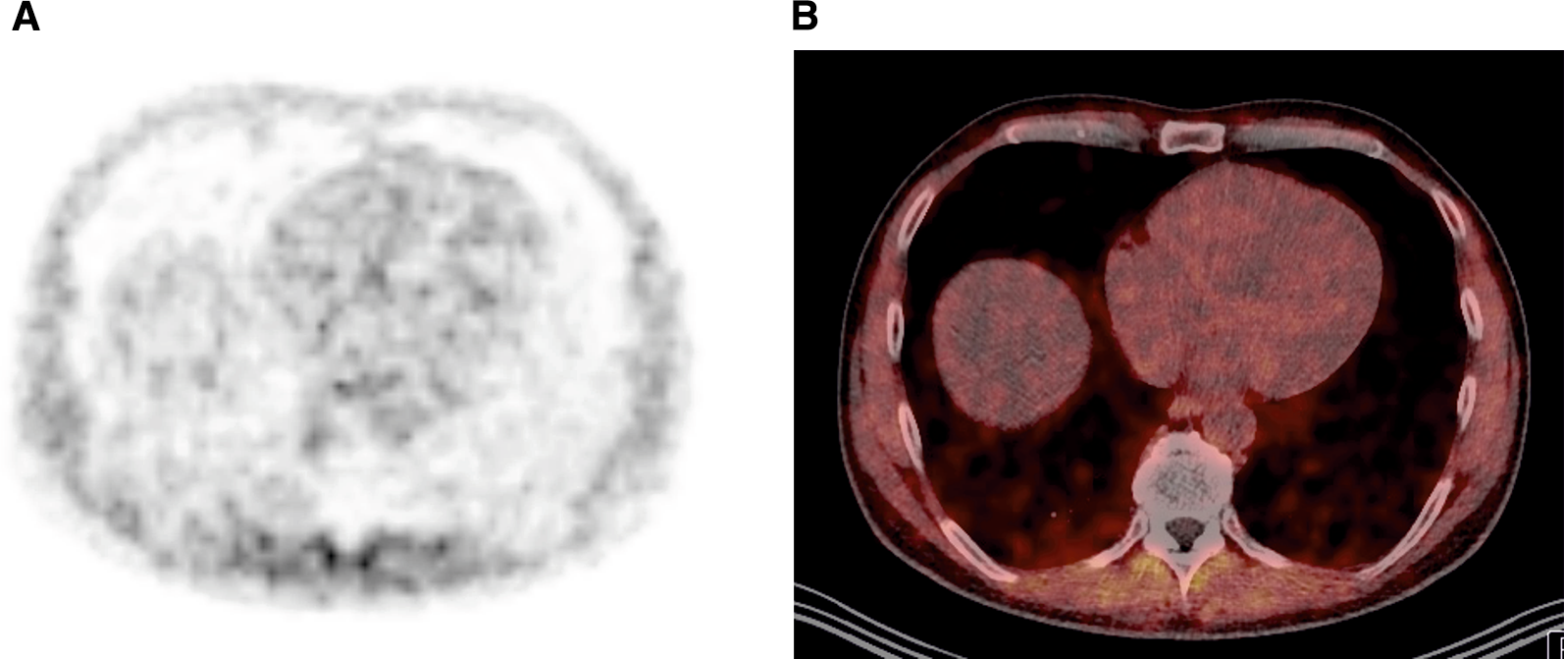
^68^Ga-FAPI-04 PET/CT Control patient. Representative reference patient without cardiac disease. No intense ^68^Ga-FAPI-04 uptake in normal myocardium was registered. A Attenuation corrected axial ^68^Ga-FAPI-04 PET. B PET/CT image fusion
